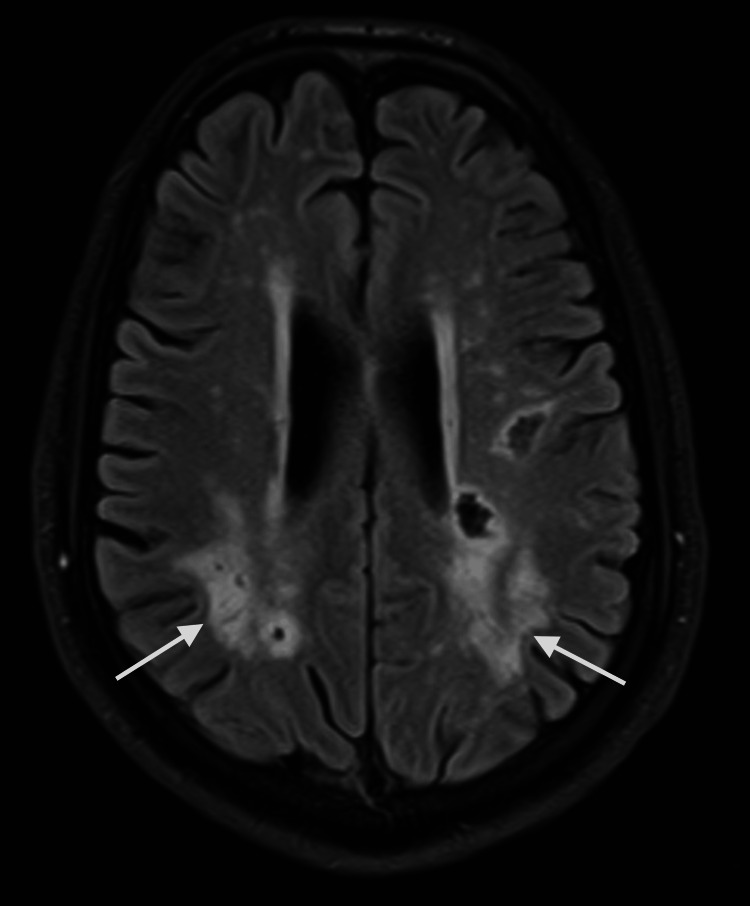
Brain MRI, FLAIR, axial view
